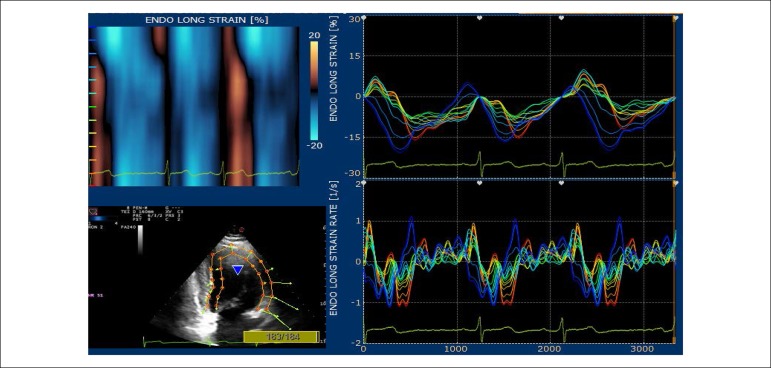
Apical two-chamber – percentage of longitudinal strain in the basal, middle and apical segments of the inferior and anterior walls.
